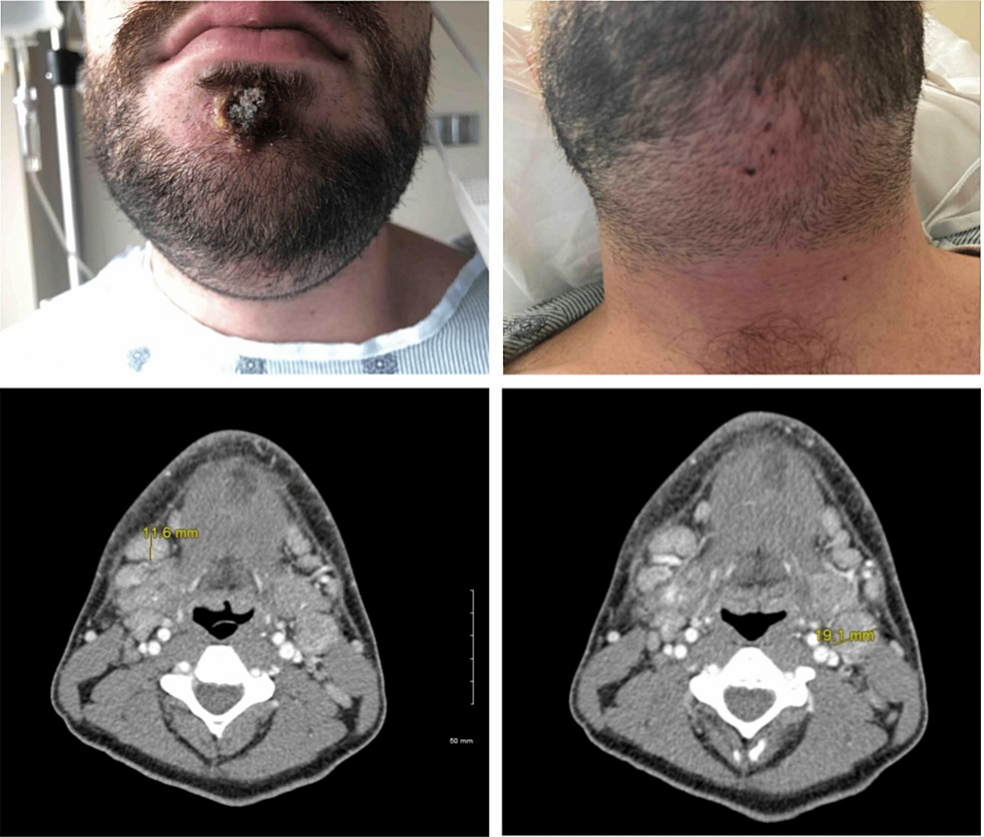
The clinical and radiographic findings of the patient's jaw swelling Left upper: A crusted 3 cm × 3 cm lesion on the patient's chin. Right upper: Diffuse swelling and erythema at the patient's jaw/neck area. Left lower: Enlarged right submandibular lymph node measuring 1.2 cm on the axial view of the patient's CT neck. Right lower: Enlarged left station IIA lymph node measuring 1.9 cm on the axial view of the patient's CT neck.
